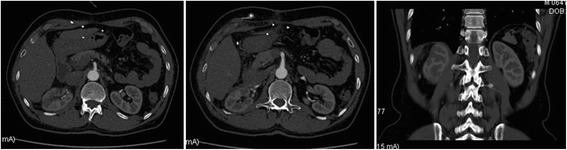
CT angiogram postoperative
